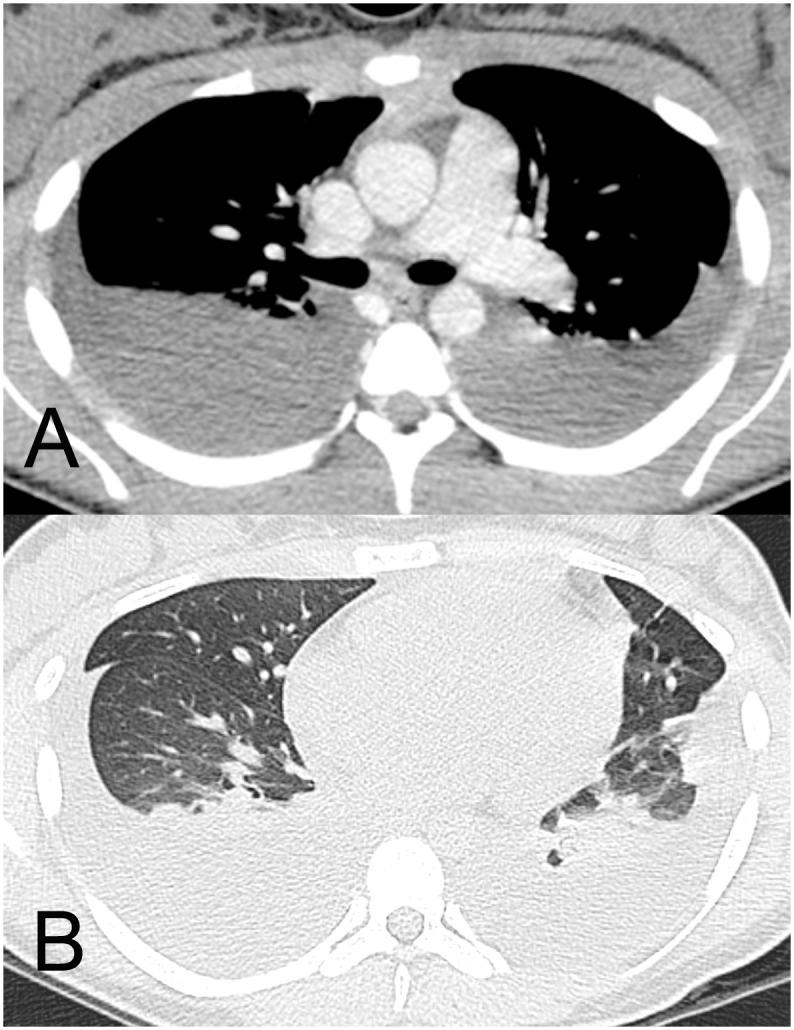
A 16-year-old girl with a confirmed diagnosis of dengue and reduced exercise tolerance and breathlessness. CT images show large bilateral pleural effusion (a, mediastinal window) and no abnormality of the lung parenchyma other than compressive atelectasis (b, lung window).
